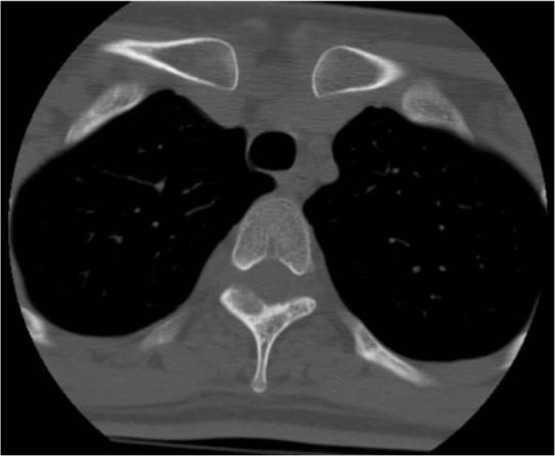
Multiple detector computed tomography image
